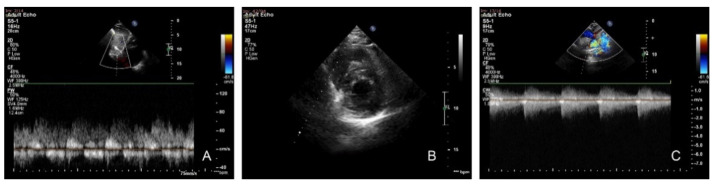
Transthoracic echocardiography. (A) Subcostal sagittal view: pulsed Doppler flow pattern in abdominal aorta—systolic waveform amplitude is low with the persistence of gradient in diastole. (B) Parasternal short axis: concentric left ventricular hypertrophy. (C) Suprasternal view: continuous-wave Doppler interrogation of the descending aorta. Note the high-velocity systolic amplitude (4.62 m/s) and maximum gradient of 85 mmHg with continuous antegrade flow through diastole.
